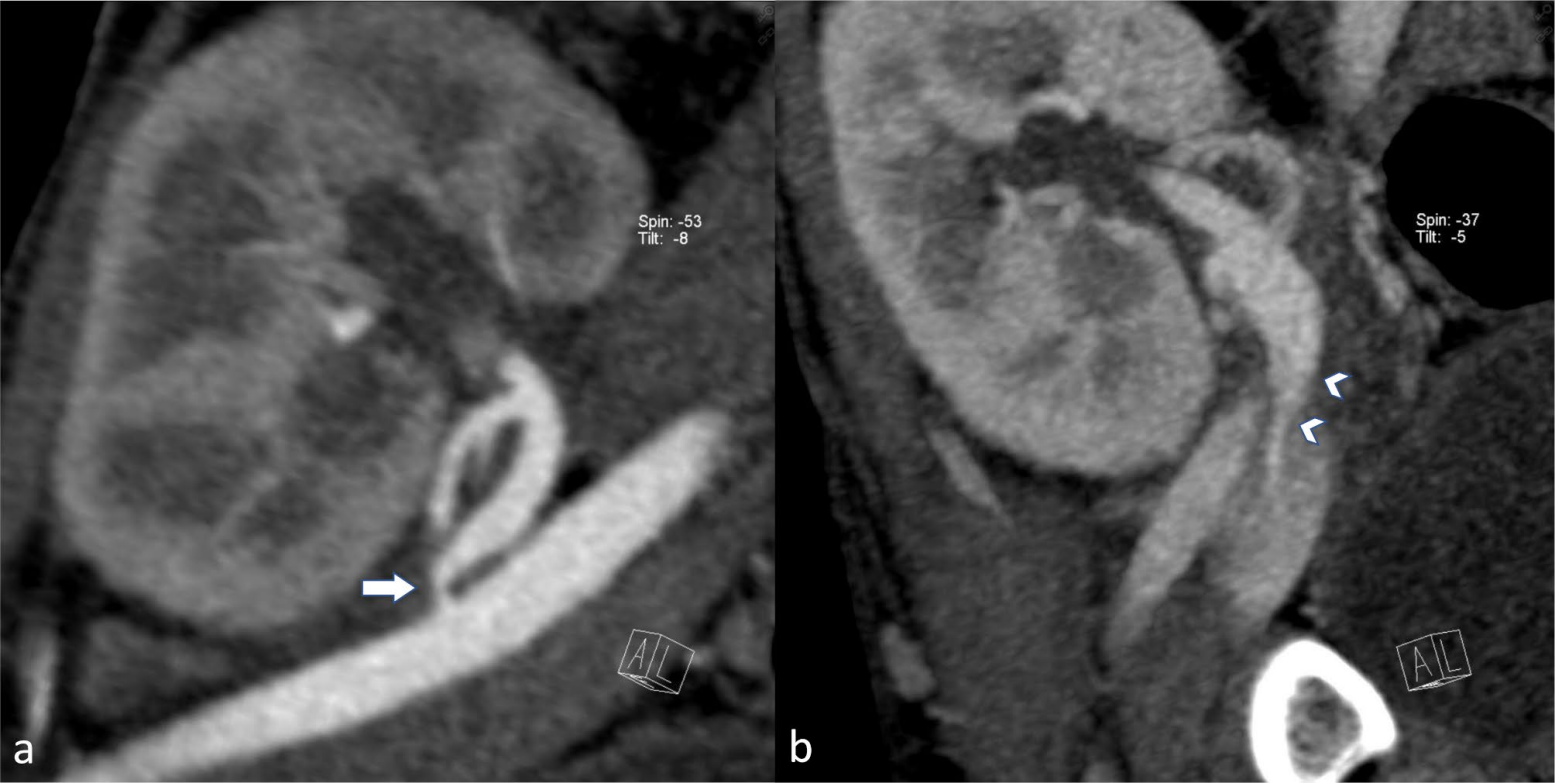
a: CTA graft artery stenosis [arrow]. b: CTA graft vein stenosis [arrowheads]
